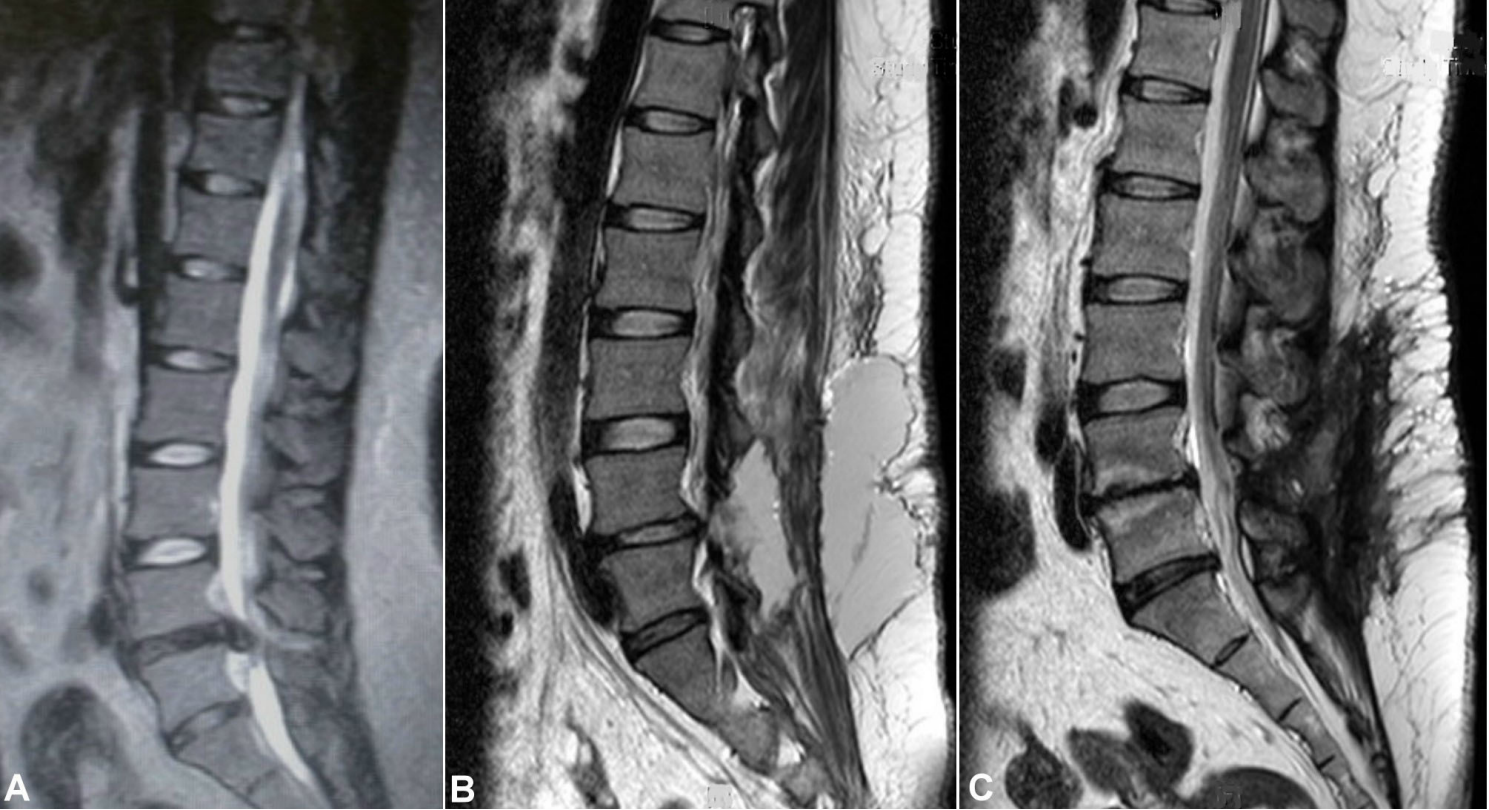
A giant pseudomeningocele noted after L4-L5 discectomy. (A) A 26-year-old obese female, body height of 170 cm, body weight 110 kg, and a BMI of 38.1 kg/m^2^. She underwent L4--L5 laminotomy and discectomy for lumbar disk herniation. (B) She developed a postoperative giant lumbar pseudomeningocele (length, 9 cm; width, 8 cm; depth, 7 cm). (C) By extirpation of the cyst, dural repair and subarachnoid drainage, the pseudomeningocele was successfully treated and confirmed on MRI at 3-month follow-up.
